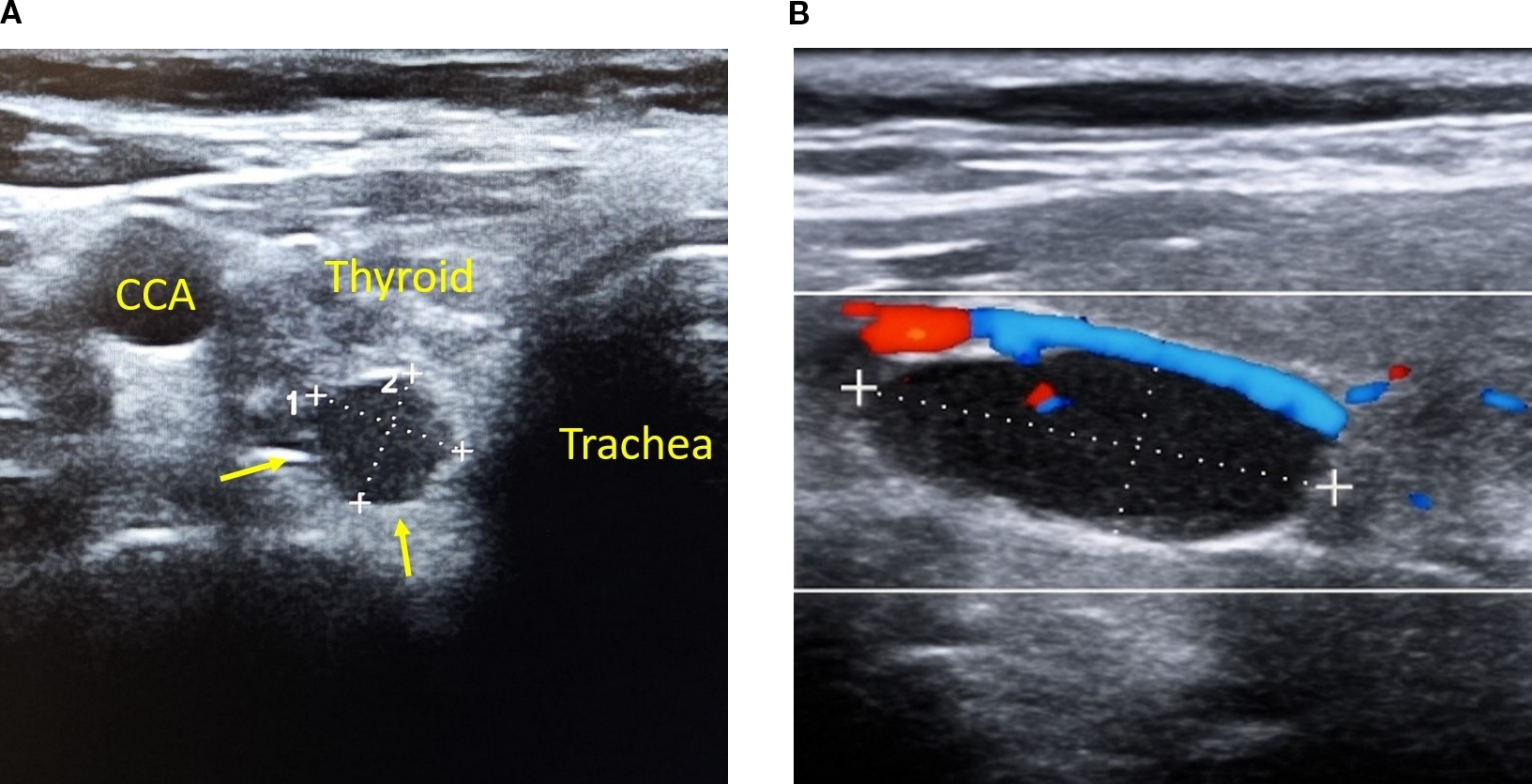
Typical ultrasonographic appearance of a parathyroid adenoma. (A) A hypoechoic, well-circumscribed lesion (yellow arrows) adjacent to the posterior aspect of the thyroid gland represents a right inferior parathyroid adenoma. Note the proximity to the common carotid artery (CCA) and trachea, labeled for anatomical reference. The lesion is located in a typical orthotopic position and demonstrates characteristic features of parathyroid adenomas on B-mode ultrasonography, including an oval shape and well-defined margins. (B) A well-defined, hypoechoic lesion representing a parathyroid adenoma is shown on color Doppler imaging. Doppler evaluation reveals a characteristic polar vascularization pattern, with feeding vessels entering from the superior pole and forming a peripheral vascular arc around the lesion. This vascular pattern is commonly observed in parathyroid adenomas and helps differentiate them from adjacent thyroid nodules and cervical lymph nodes, which typically exhibit different vascular profiles.
